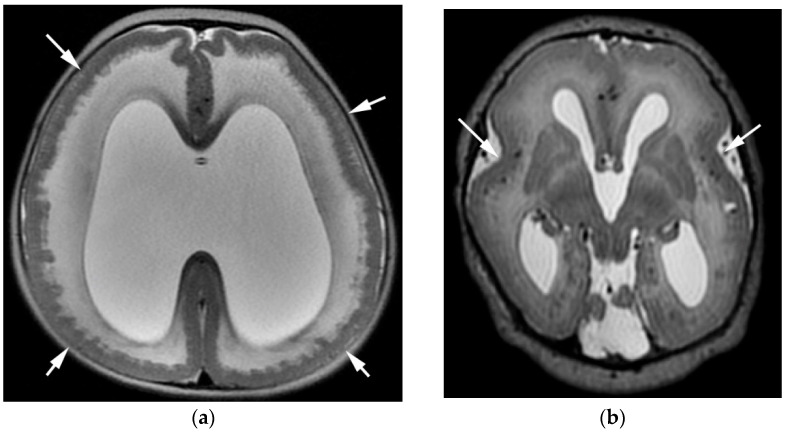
(a) Axial T2-weighted image of cobblestone cortex with a polymicrogyria phenotype (due to small gaps in pial limiting membrane). Note the simplified gyral pattern (white arrows). (b) Axial T2-weighted image of cobblestone cortex with a lissencephalic appearance, due to larger gaps in the pial limiting membrane. Note the rudimentary sylvian fissures (white arrows).
